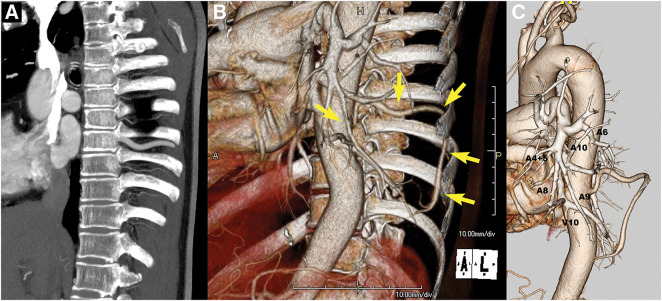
(A) Sagittal view of contrast-enhanced chest CT shows an abnormally thick 9th intercostal artery. (B) A 3D reconstruction image, overlapping surrounding structures such as the thoracic wall, shows the thick 9th intercostal artery branching from the descending aorta and an anomalous artery branching into the thoracic cavity (yellow arrows). (C) A 3D reconstruction focusing on the pulmonary and aortic systems shows almost normal branching of the left pulmonary artery to A6, A8, and A9. However, A10 demonstrates hypoplasia. While V10 is abnormally thick and well-developed, no vascular malformations, such as abnormal anastomoses or arteriovenous fistulas, are observed between the anomalous artery originating from the 9th intercostal artery and the pulmonary venous system.
